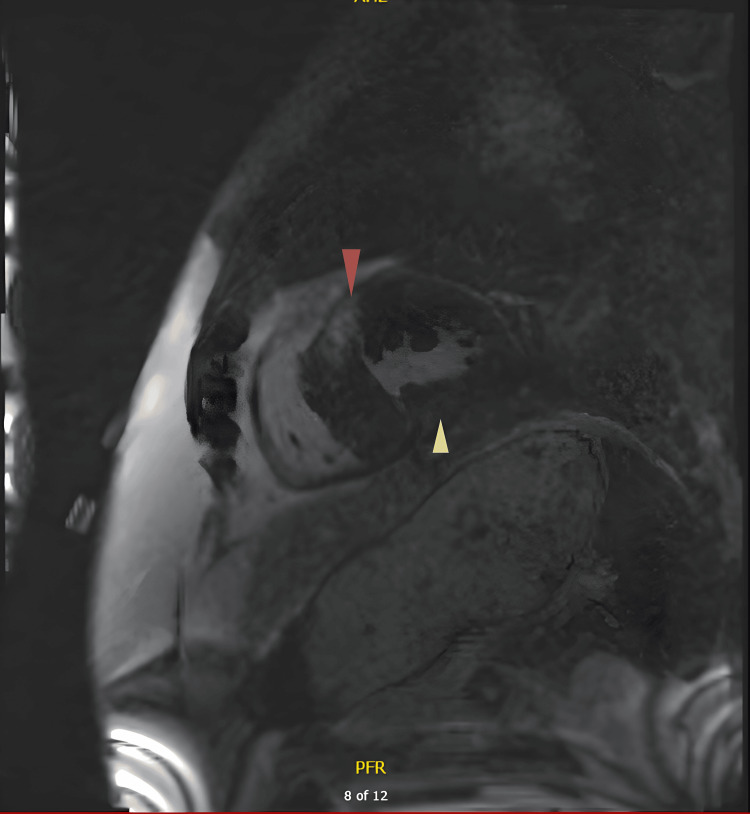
Cardiac magnetic resonance imaging - short-axis view at the papillary muscle level Patchy mid-wall late gadolinium enhancement (red and yellow arrows) at mid anteroseptum and inferoseptum, indicating myocardial fibrosis.
